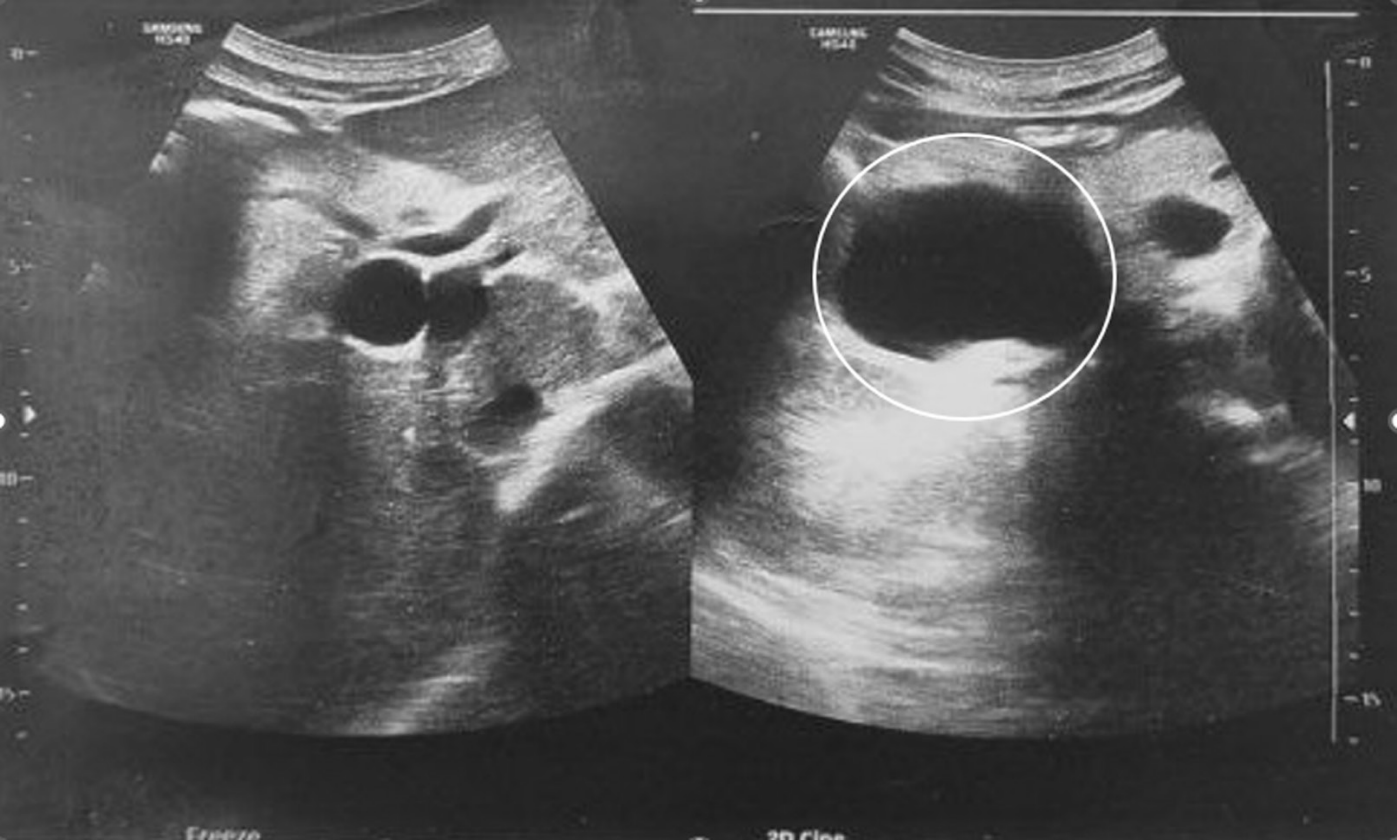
Endoscopic Ultrasonography suggested a large cystic thick wall lesion at the pancreatic head area. (white circle).
